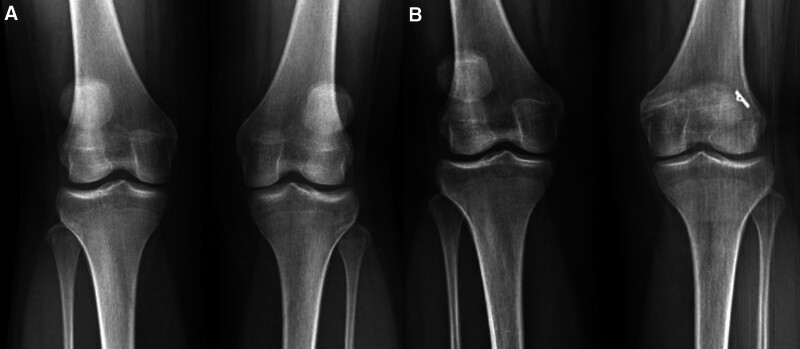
Preoperative (A) and postoperative (B) anterior-posterior X-ray graphics of the patient who underwent MPFL reconstruction. MPFL = medial patellofemoral ligament.
